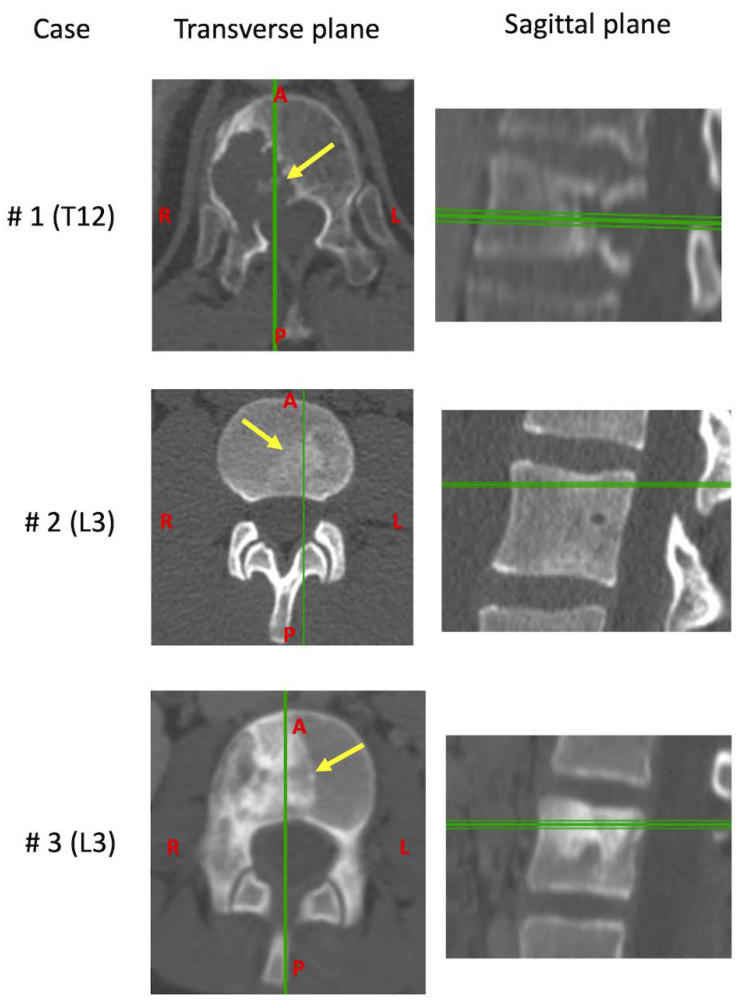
Transverse and sagittal planes of the CT scans for each vertebra before the resection. The arrows indicate the position of the tumor, the vertical and horizontal lines indicate the relative position of the plans.
